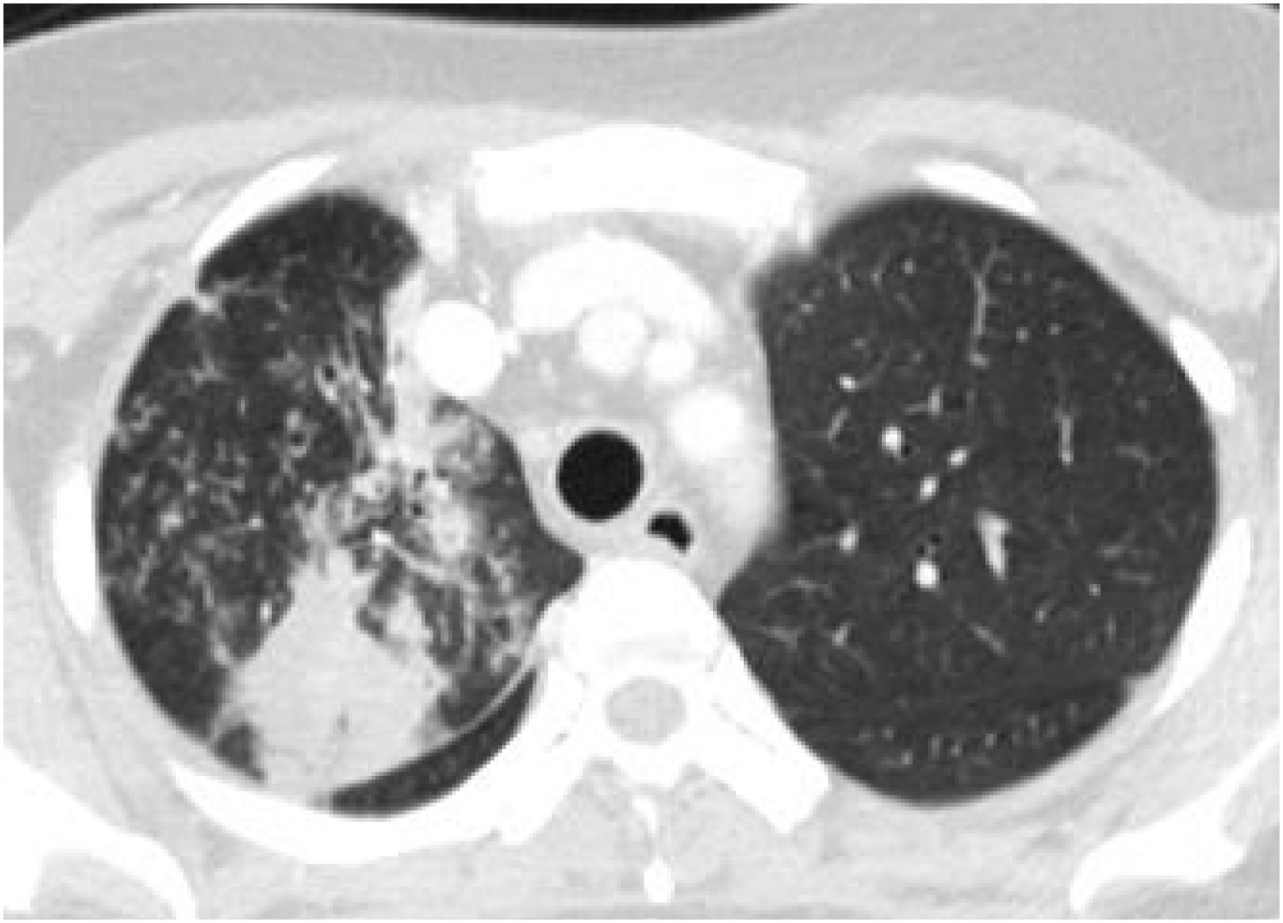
A 37-year-old woman with pulmonary TB with positive sputum MTB/RIF. Sputum acid-fast bacilli was negative, but sputum Xpert MTB/RIF was positive. Chest CT shows consolidation with multiple centrilobular nodules in the right upper lobe. Rapid TB medication was initiated and sputum culture also showed positive later.
